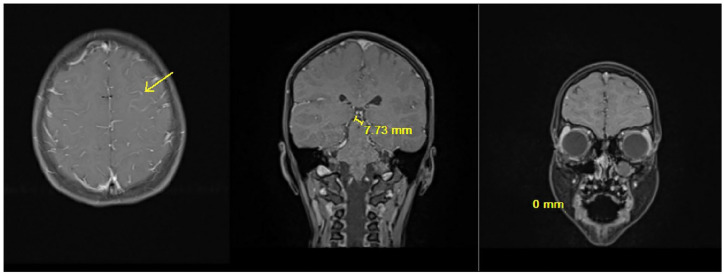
MRI Brain hospital day 5 new mild cortical reduced diffusion and edema along the left superior frontal and precentral sulci. Additional very mild edema along the left gyrus rectus. Appearance likely reflects mild ischemic injury or focal cerebritis.
